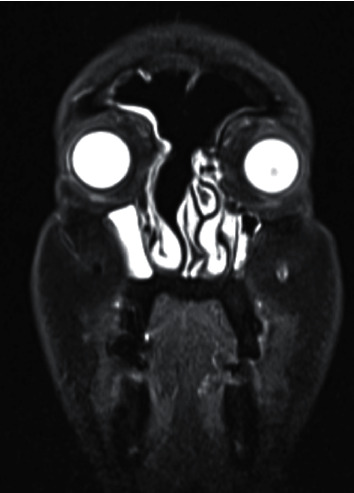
Post-op MRI scan (coronal plane). Postoperative MRI T1 with gadolinium enhancement after 6 months of follow-up. Total tumour clearance and no evidence of residuum/recurrence are confirmed.
